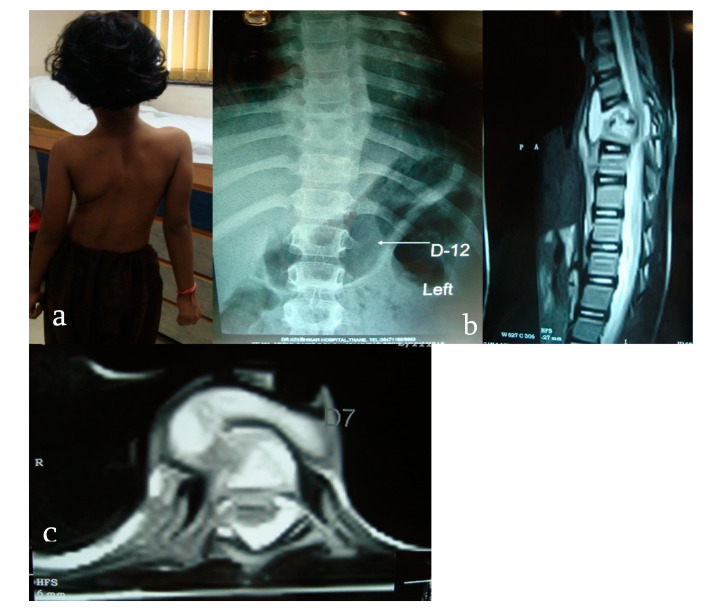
(a) A 6-year-old girl presents with scoliosis noticed by parents 4 months previously. (b) X-ray of the dorsal spine showing collapse of the D8 vertebra and a fusiform-shaped soft tissue shadow suggestive of an abscess. (c) MRI of the dorsal spine showing collapse of the D8 vertebral body with marrow edema, prevertebral and paravertebral collection with subligamentous and epidural extension, and epidural involvement (canal encroachment).
